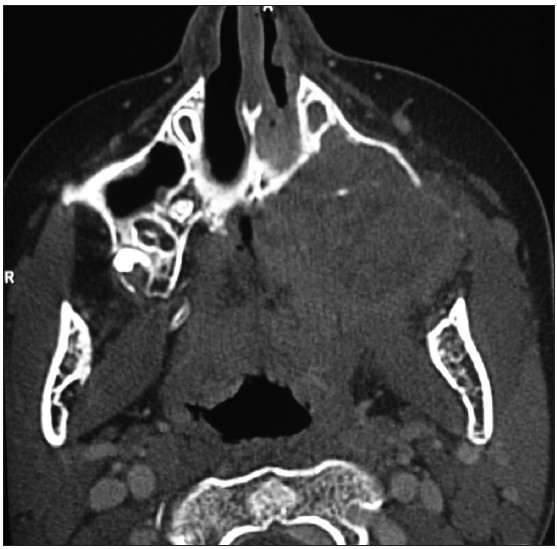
CT scan of the patient in Figure 1 showing extensive destruction of maxillae
